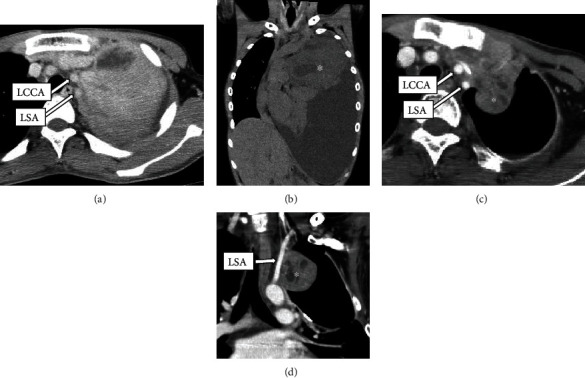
Before initial operation: (a) horizontal view, (b) frontal view. Chest computed tomography (CT) radiograph showing a left superior mediastinal tumor (^∗^) measuring 123 × 120 × 88 mm in size with massive pleural effusion. Before third operation: (c) horizontal view, (d) frontal view. Follow-up chest CT revealing local recurrence with rapid growth in the left superior mediastinum. The tumor had attached to the left common carotid artery (LCCA) and the left subclavian artery (LSA).
